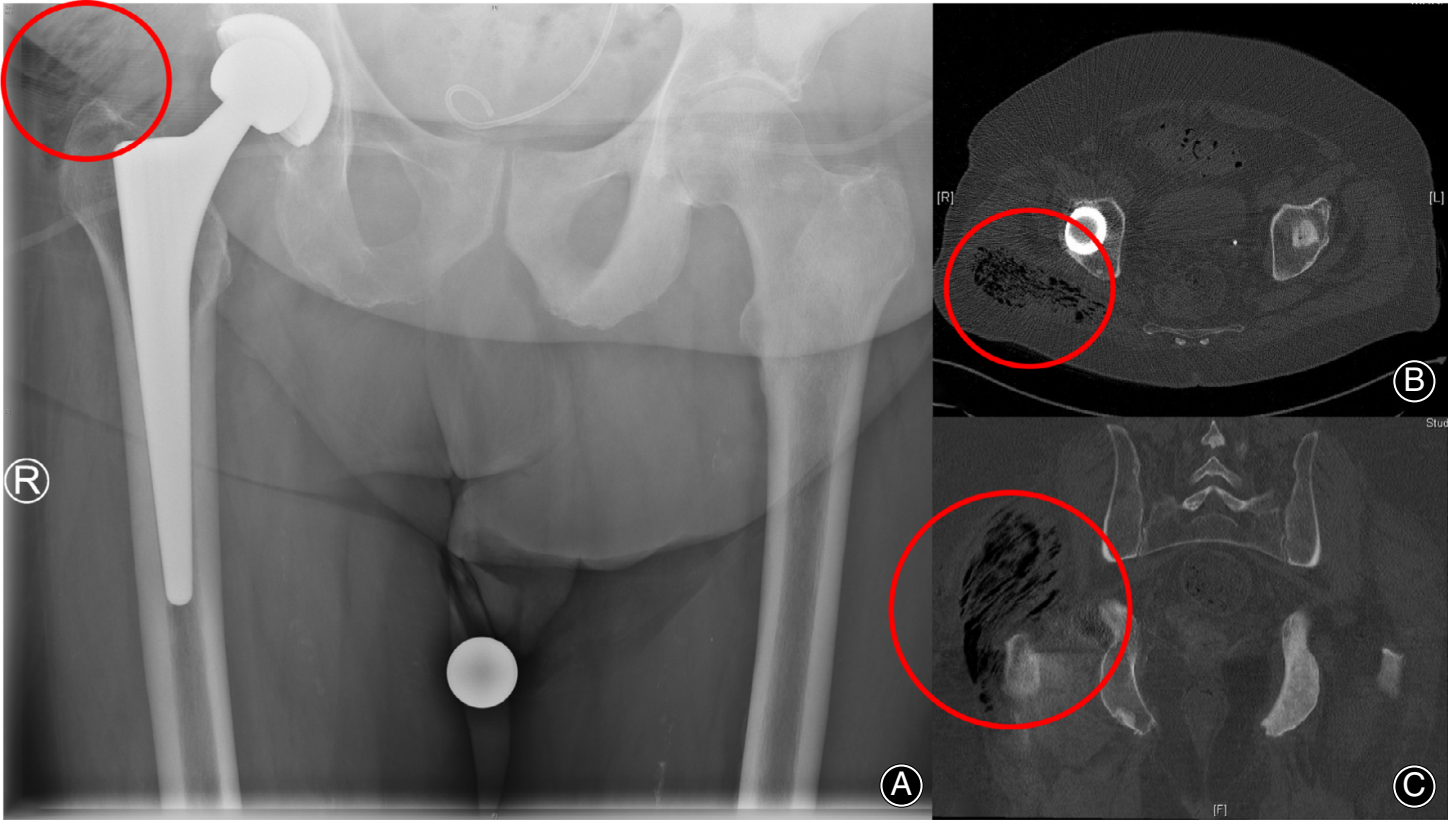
X‐ray of the pelvis of a patient with clostridial gas gangrene caused by *Clostridium septicum* complaining about pain in his right hip. The red circle marks gas in the musculus gluteus (A). CT scan shows the extent of gas formation (red circle) (B, C).
